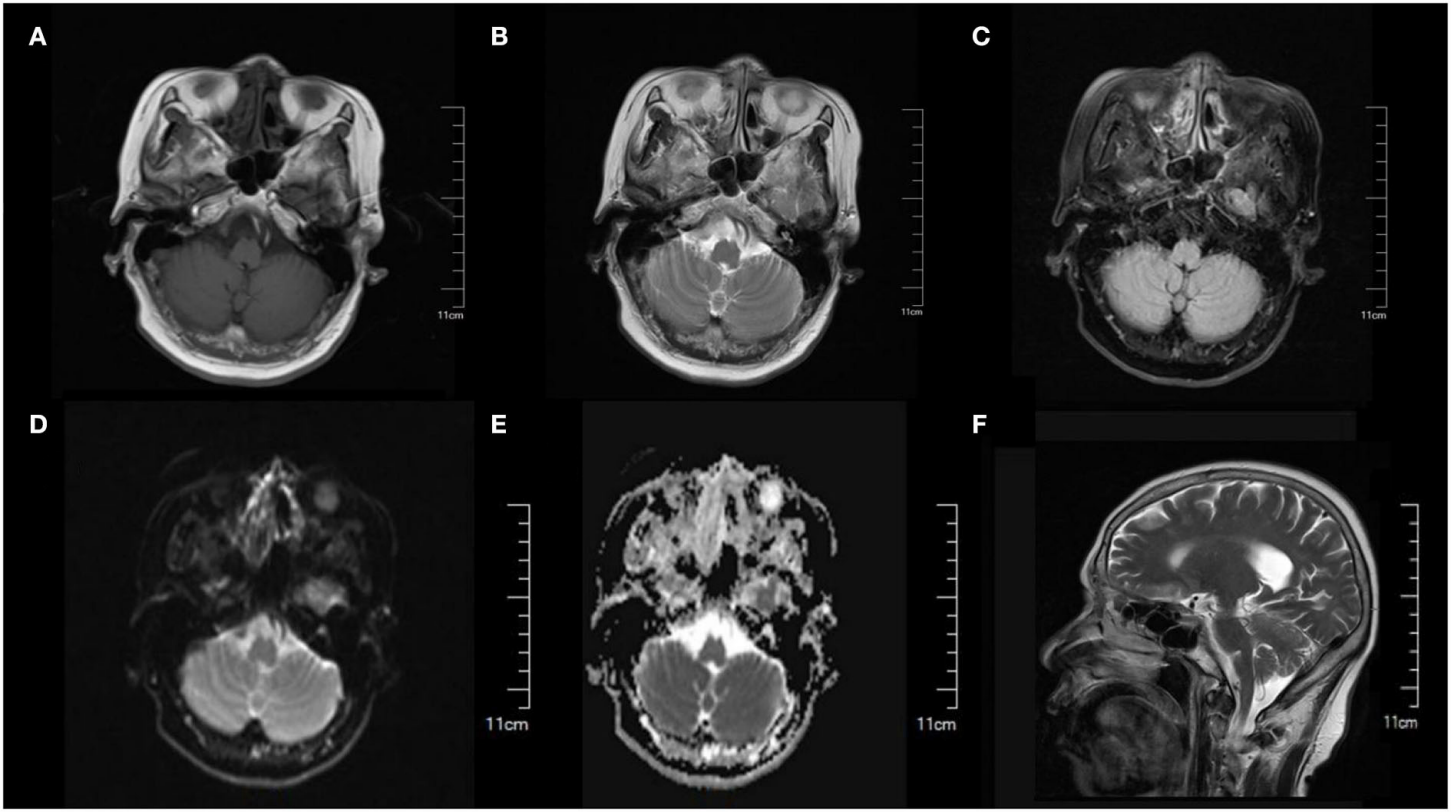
Cranial MRI showed normal brain structure and no obvious abnormal signal was found. (A) T1-weighted image. (B) T2-weighted image. (C) FLAIR image. (D) DWI image. (E) ADC image. (F) Sagittal section image.
